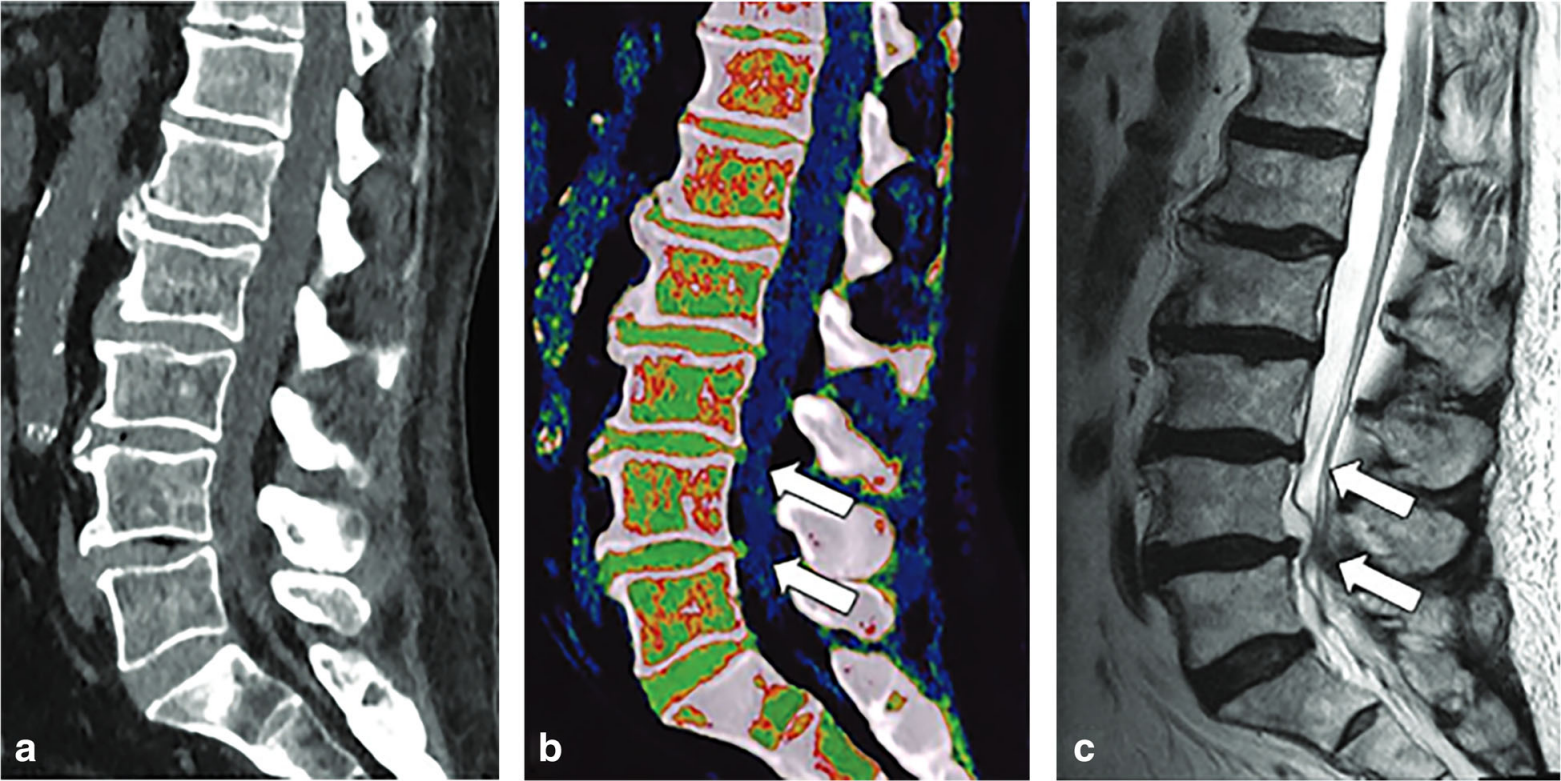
Spine dual-energy computed tomography. Standard grayscale series (a) shows typical findings of spondylarthrosis with vacuum phenomena in L3/L4 and L4/L5 intervertebral discs. Virtual non-calcium reconstruction with optimization for intervertebral disc analysis (b) can finely show the protrusion of lumbar discs (*arrows*), confirmed by magnetic resonance imaging T2-weighted sequence (c) (*arrows*). From Booz et al. [34]
